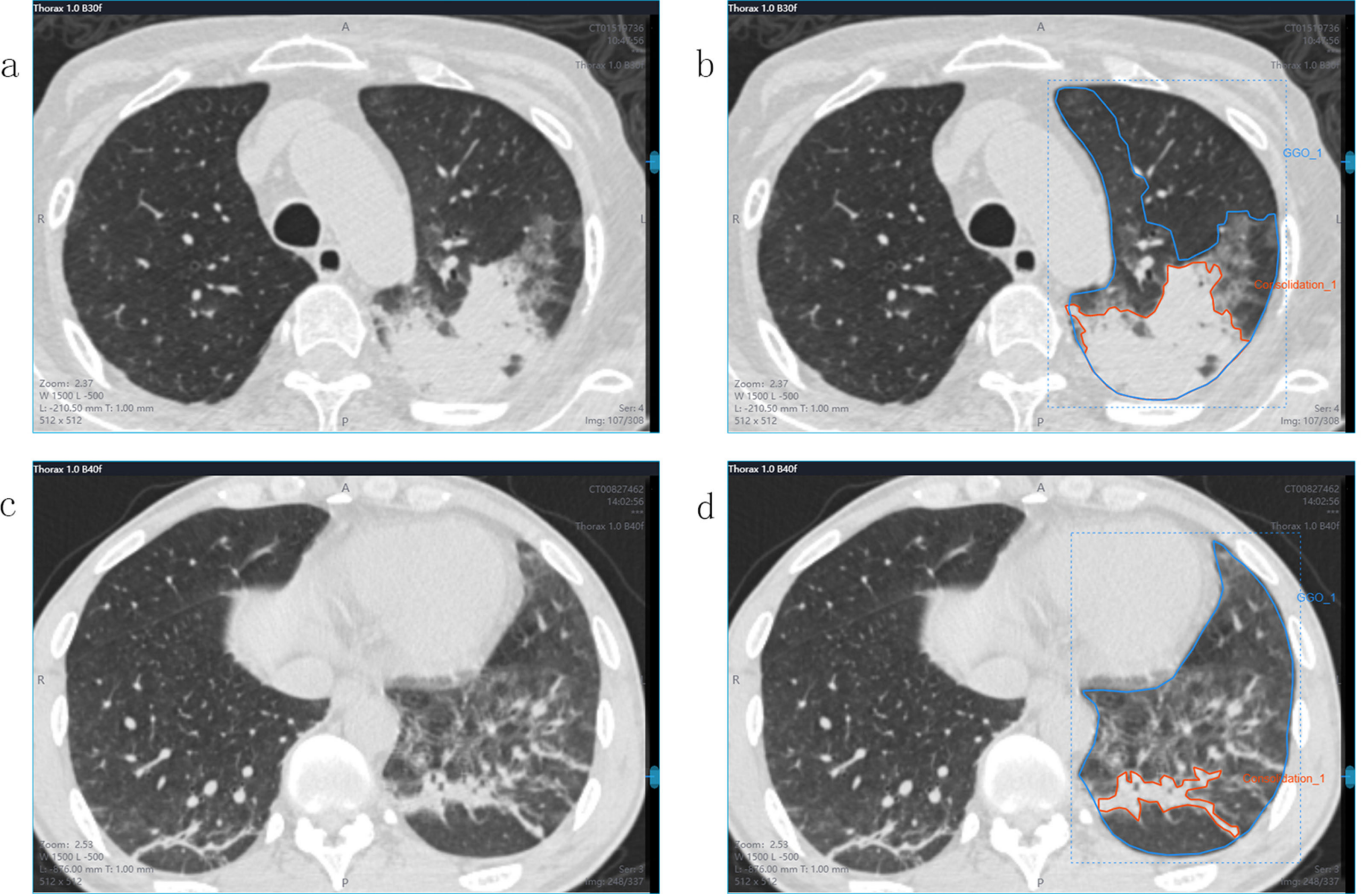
CT images and ROI segmentation. Panels (a, b) showed a 55-year-old male with IPA, while panels (c, d) showed a 36-year-old male with PJP. Both patients presented similar consolidations and surrounding GGO areas on CT images. panels (b, d) show the ROI segmentation method. The orange lines include only the consolidation area as the ROI. The blue lines include both the consolidation area and the GGO area as the ROI.
